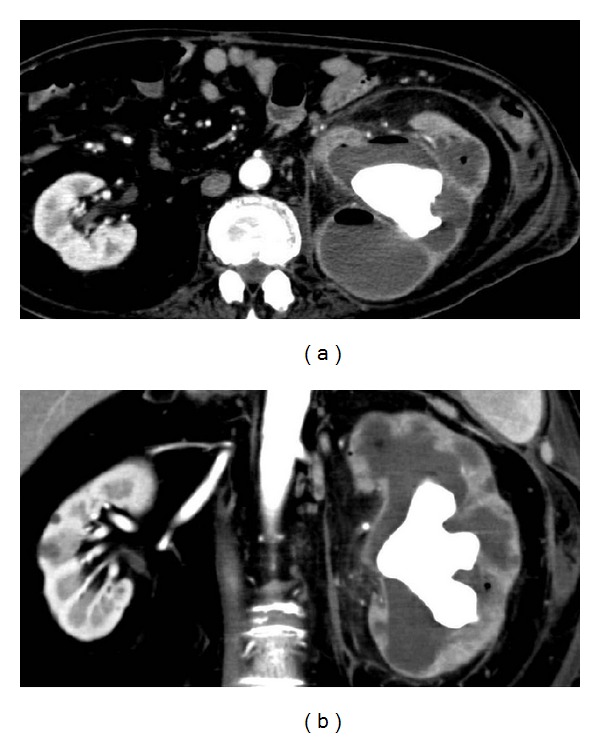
CT findings of the abdomen ((a) transverse section and (b) coronal section) CT showed an enlarged nonfunctioning left kidney and staghorn calculi, with distention of the collecting system.
